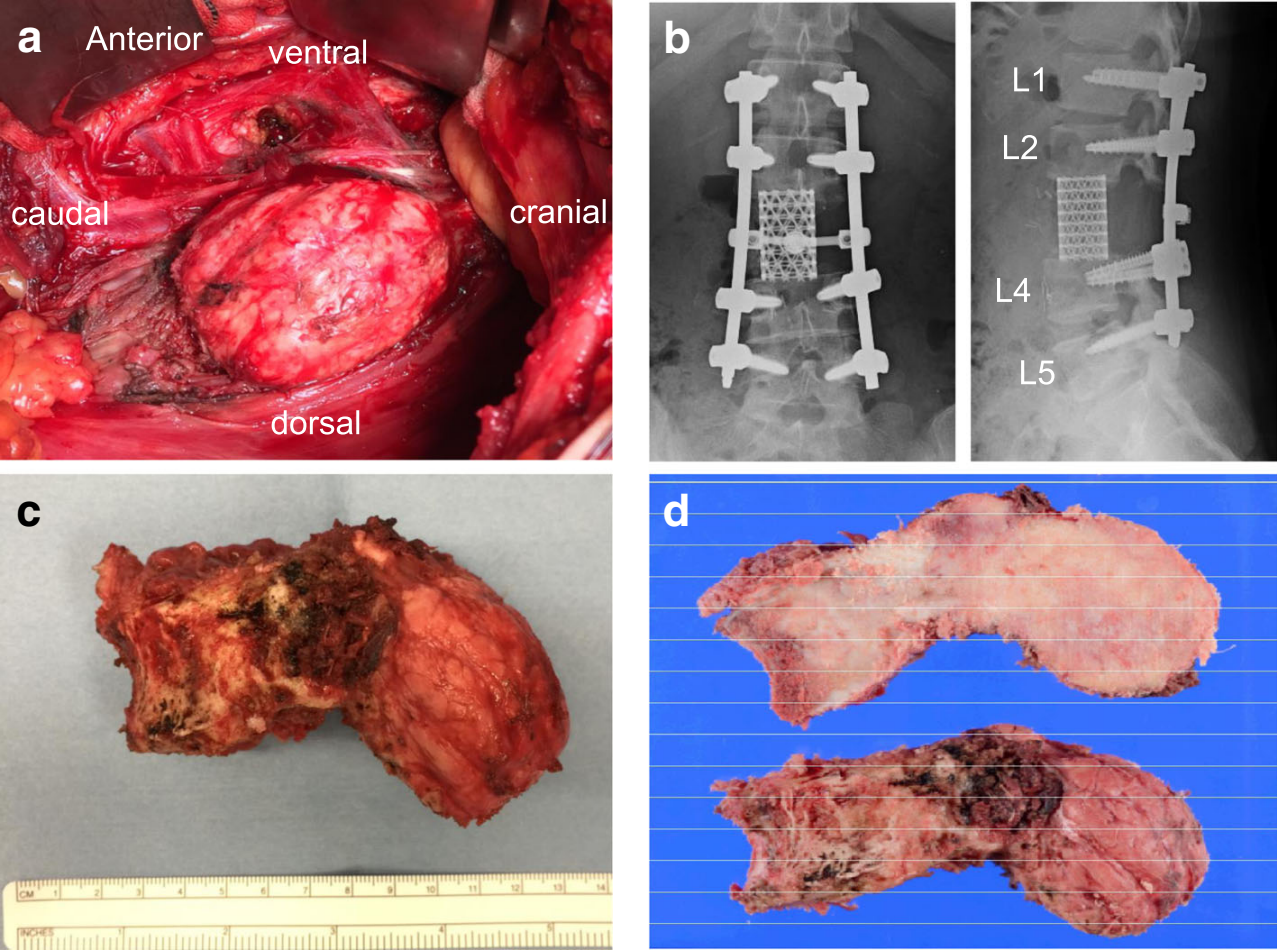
a Intraoperative examination of the tumor at anterior retroperitoneal approach. b Plain radiographs of the anteroposterior and lateral view. c, d Results showing that the L3 vertebral tumor body had been completely removed by the anterior transperitoneal approach
